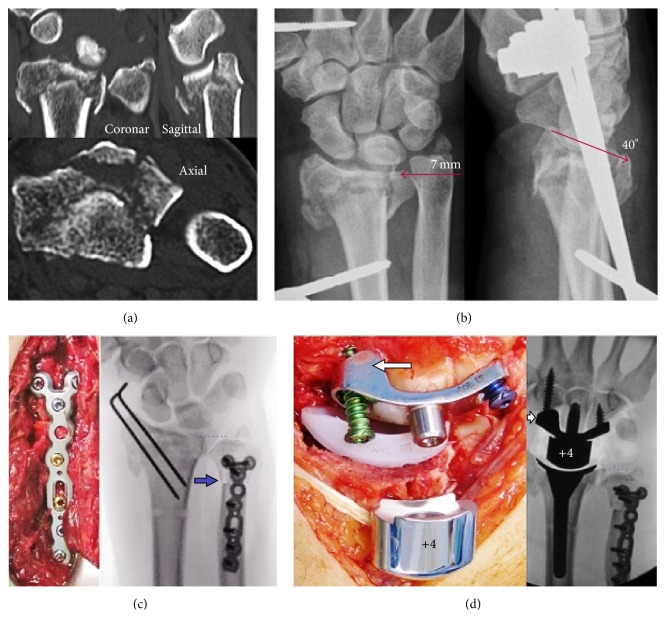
Case report 1 (preoperative and surgical procedures): (a) initial CT images in three levels; (b) AP and lateral radiographs after closed reduction and external fixation; (c) intraoperative clinical photo and AP fluoroscopy after removal of external fixateur, percutaneous pinning, and USO (*arrow*) showing no ulnar-positive variance (*points*); note the displaced ulnar styloid base fracture; (d) intraoperative clinical photo and AP fluoroscopy after Maestro WRS insertion showing correct position of implant; note the scaphoid augment of the carpal component after complete scaphoid excision (*arrow*).
